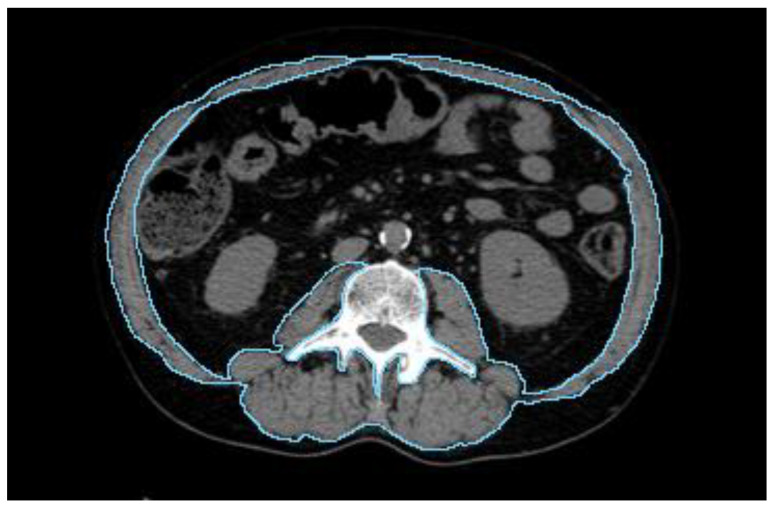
Delineation of the skeletal muscle area on a CT scan at the level of the third lumbar vertebra. Adapted from Medici F et al., 2022 [11].
